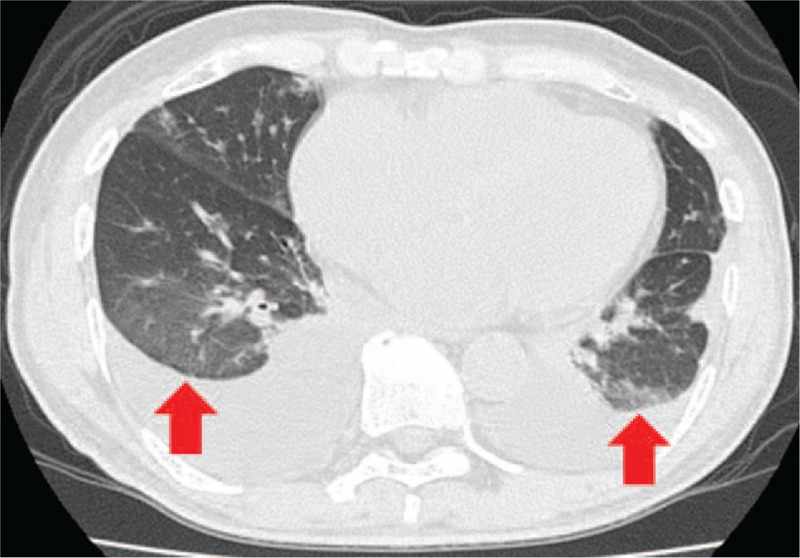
Chest CT showed peripheral ground glass opacity (arrow), dilated heart, and large amount of pleural effusion. CT = computed tomography.
